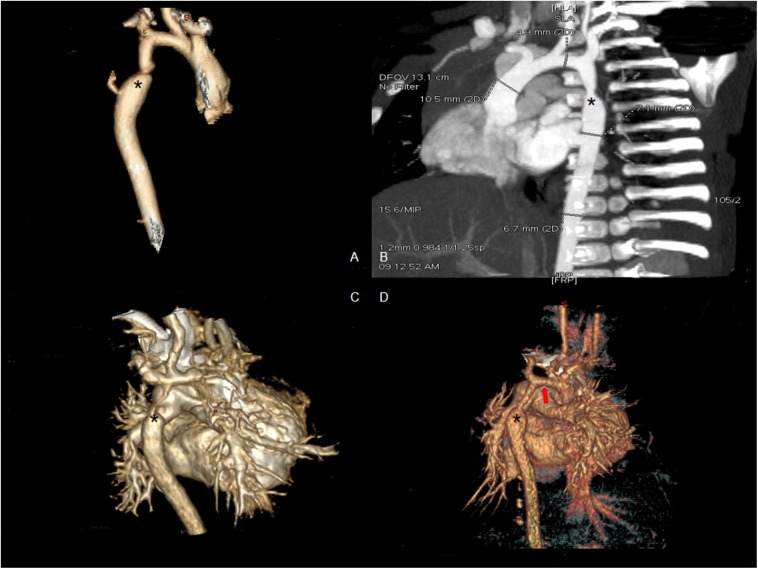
Enhanced CT scan showing coarctation of the aorta before surgery. (A,B) Eight-month male with coarctation of the aorta (asterisk shows the coarctation). (C) Four-day-old male with severe coarctation of the aorta (asterisk shows the coarctation). (D) One-month-old male with coarctation of the aorta and hypoplasia of the aortic arch (asterisk shows the coarctation and red arrow shows hypoplasia of the aortic arch).
